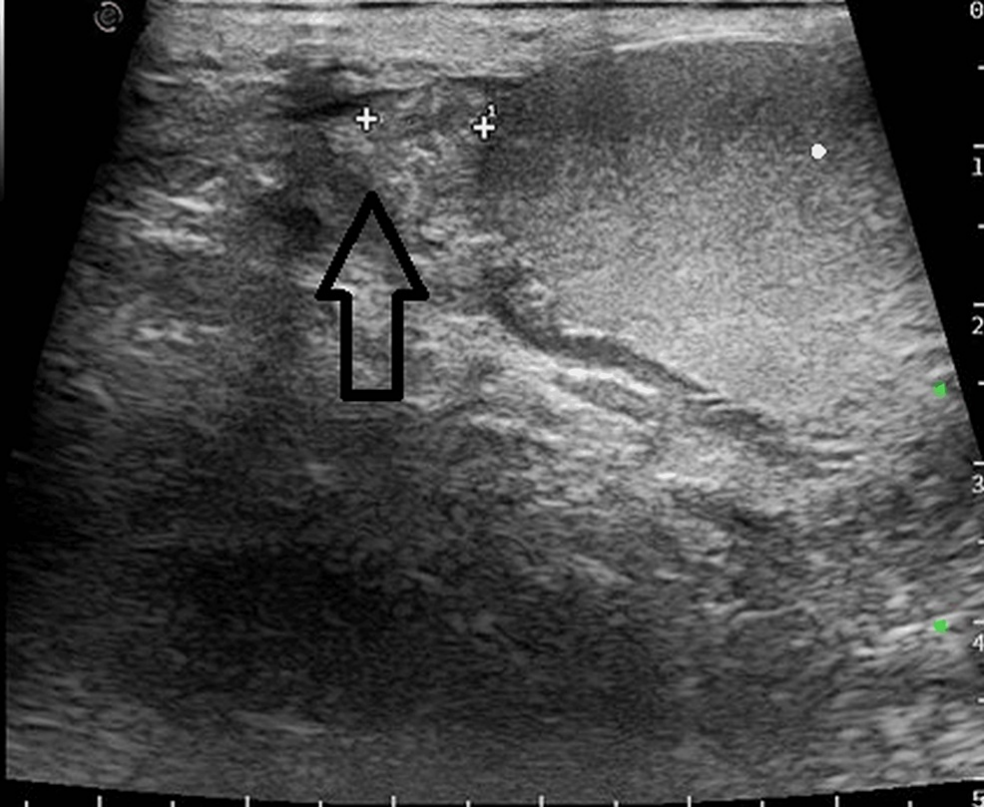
Sonography reveals normal right epididymis.
